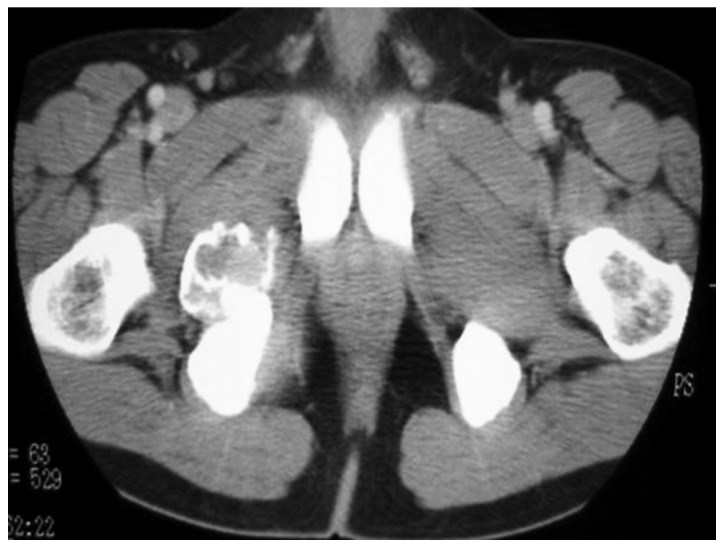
Case two: Contrast-enhanced computed tomography showing a homogeneously-enhanced mass in the pelvis.
